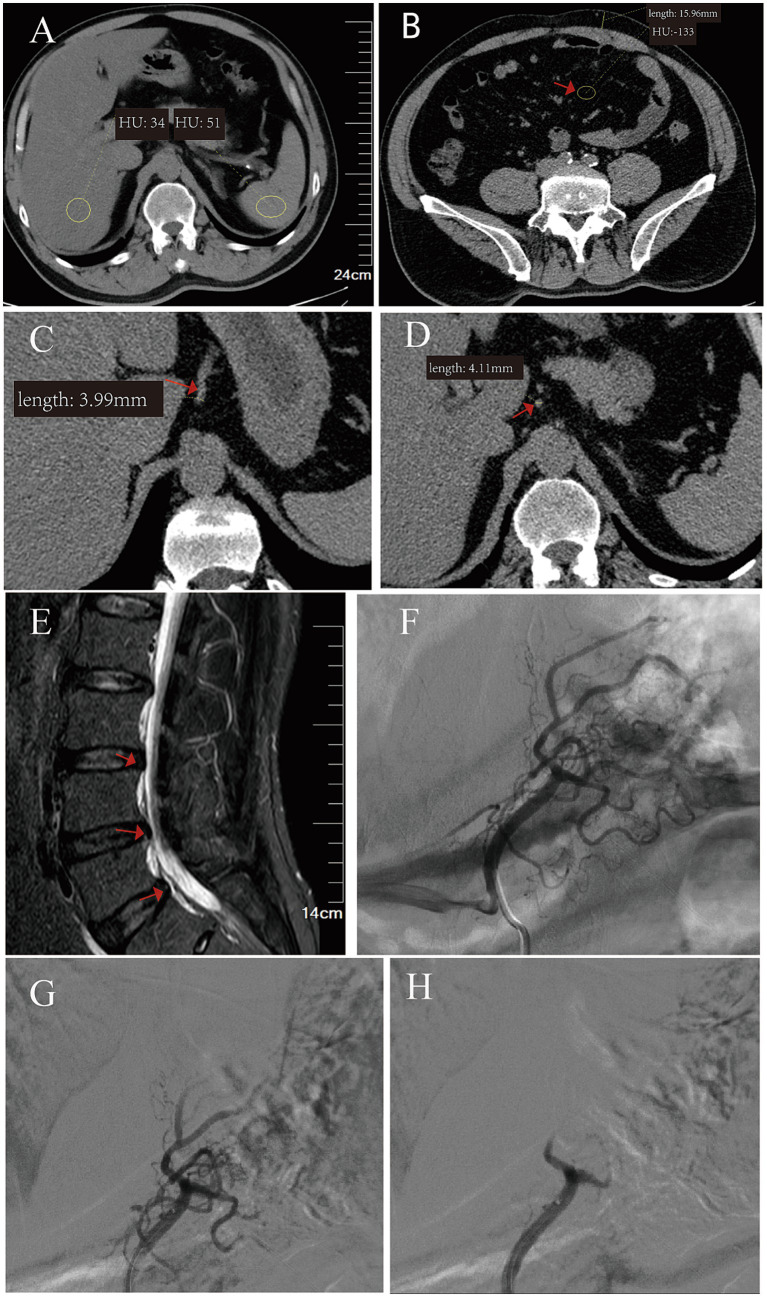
Pre- and post-procedural imaging findings of left gastric artery embolization for obesity. (A) Pre-procedure CT scan showing moderate hepatic steatosis. (B) Axial CT demonstrating increased abdominal wall fat thickness (15.96 mm) and mesenteric fat deposition (red arrow). (C,D) Coronal CT images measuring left gastric artery diameter: 3.99 mm (C) and 4.11 mm (D), confirming suitability for superselective embolization. (E) Sagittal MRI revealing lumbar disc herniation at L4/5 and L5/S1 levels (red arrows). (F) Pre-embolization digital subtraction angiography: an enlarged left gastric artery with abundant fundal branches supplying the gastric body. (G) Post-embolization DSA demonstrating complete occlusion of the terminal branches. (H) Post-procedural DSA confirming the absence of residual flow in the main trunk.
